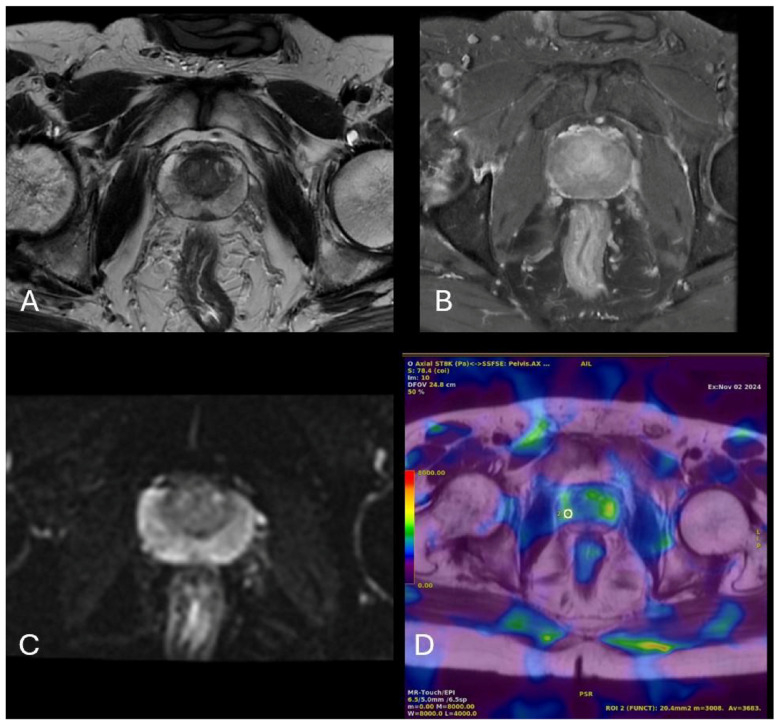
MRI and MRE findings of a 43-year-old patient presenting with dysuria, polyuria, and nocturia and a PSA level of 2.75 ng/mL. Axial T2-weighted imaging shows mild signal loss in the peripheral zone of the prostate (A), while no significant contrast enhancement is observed in the prostate parenchyma following intravenous contrast administration (B). Additionally, no diffusion restriction was detected on diffusion-weighted imaging (C). In the corresponding MRE image (D), a region of interest (ROI; white open circle) was placed over a yellow-green coded area representing the stiffest region within the parenchyma, yielding a stiffness value of 3.683 kPa.
